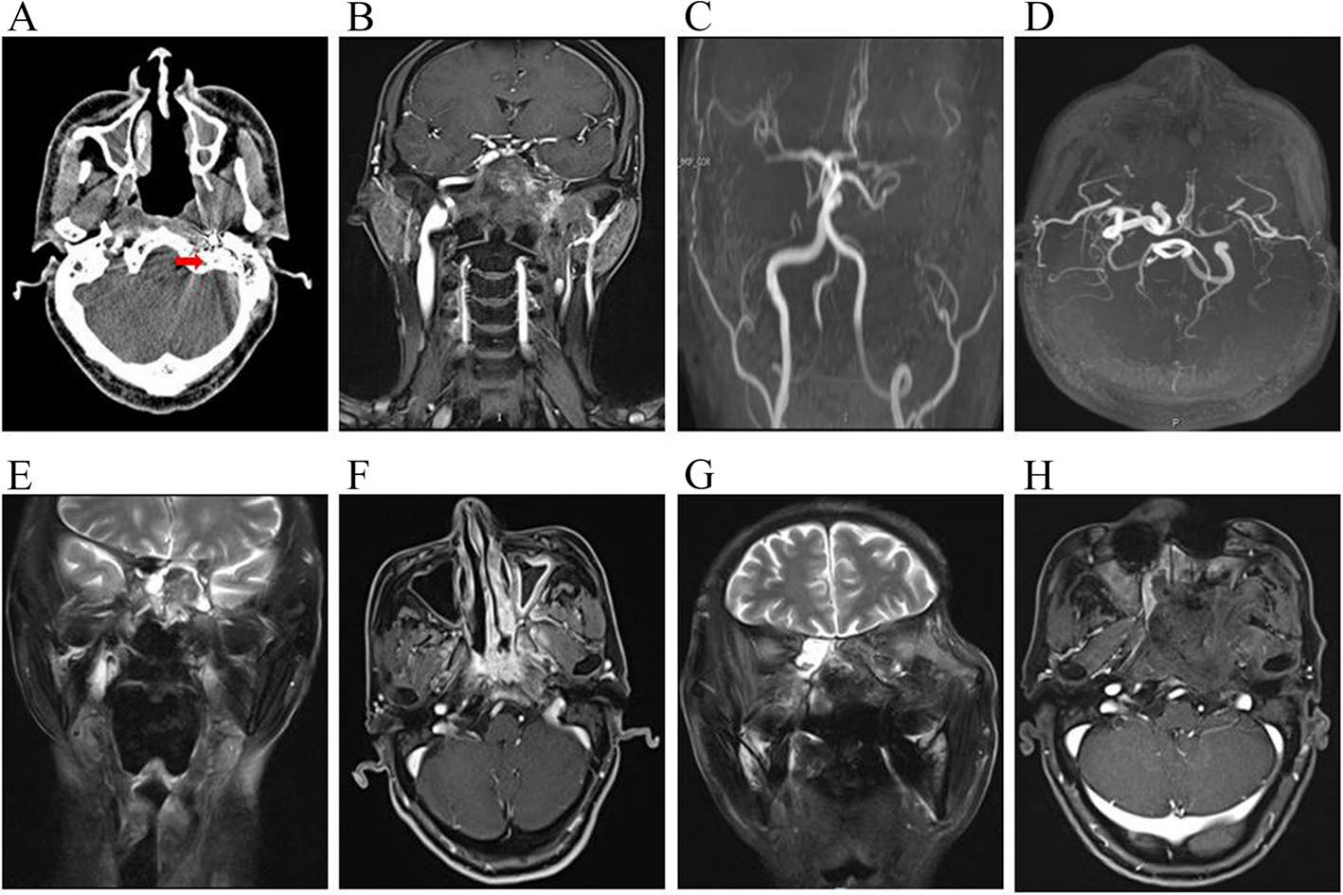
Imaging results of ICA embolization. A Horizontal enhanced CT demonstrates a diffuse soft tissue in the left nasopharyngeal lateral wall, parapharyngeal space and retropharyngeal area. Radial artifacts of high density martix coils were noted (arrow). B Coronal enhanced MRI demonstrates enhanced nodules on the left nasopharyngeal lateral wall involving the carotid sheath space and middle skull base bone. C and D MRA shows no development of the left ICA. The left middle cerebral artery is slightly thinner than the contralateral arttery, but the cerebral artery ring is generally intact. Enhanced MRI coronal T2 (E) and horizontal T1 (F) images showed soft tissue lesions on the posterior wall of the nasopharyngeal top and the left lateral wall, involving bilateral posterior pharyngeal head longus muscle, left choanal, pteryopalatine fossa, bilateral middle skull base bone and sphenoid sinus. The left internal carotid artery showed post-embolization changes. Enhanced MRI coronal T2 (G) and horizontal T1 (H) images demonstrated that the original lesions in the left nasopharynx and middle cranial fossa had been resected. CT: computed tomography; ICA: internal carotid artery; MRI: magnetic resonance imaging; MRA: magnetic resonance angiography
